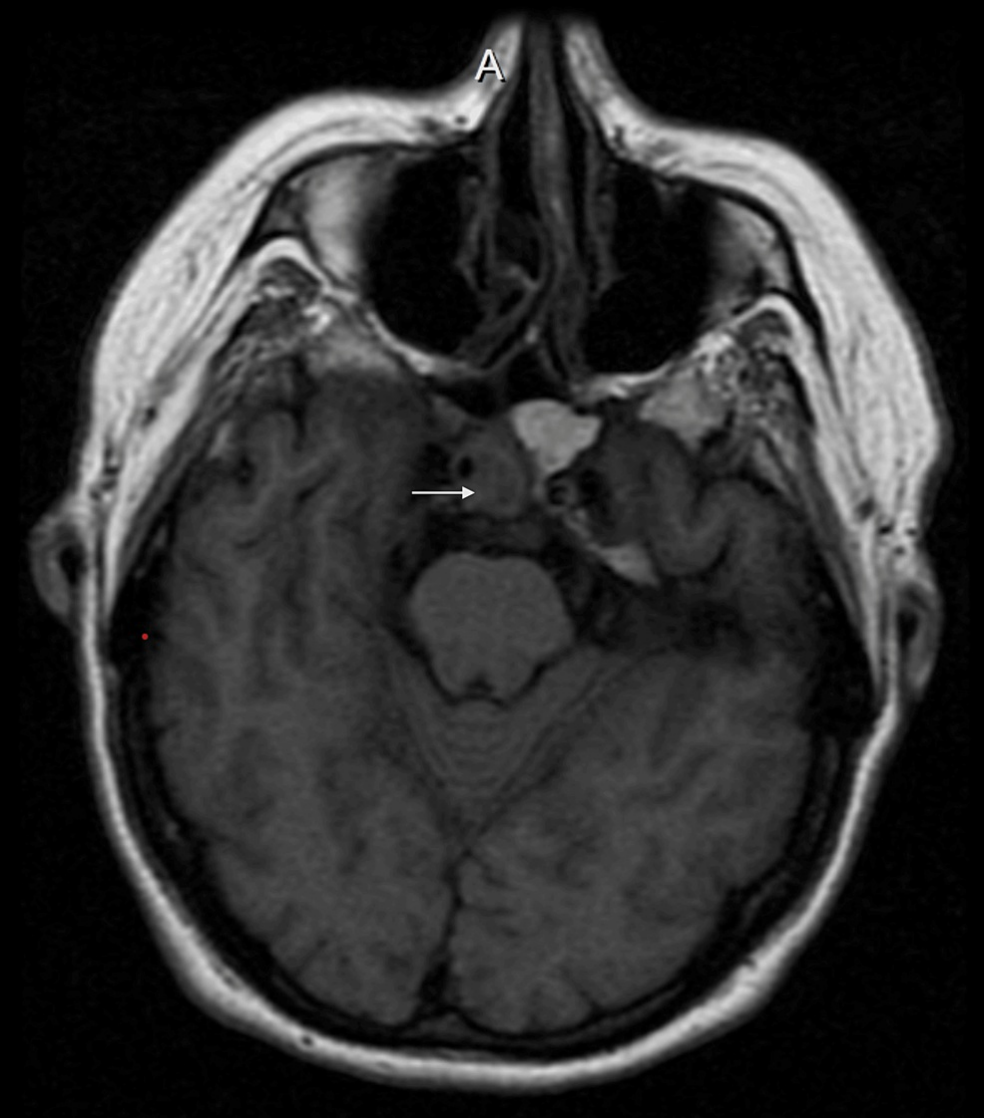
MRI brain showing opacification in bilateral sphenoid sinuses
